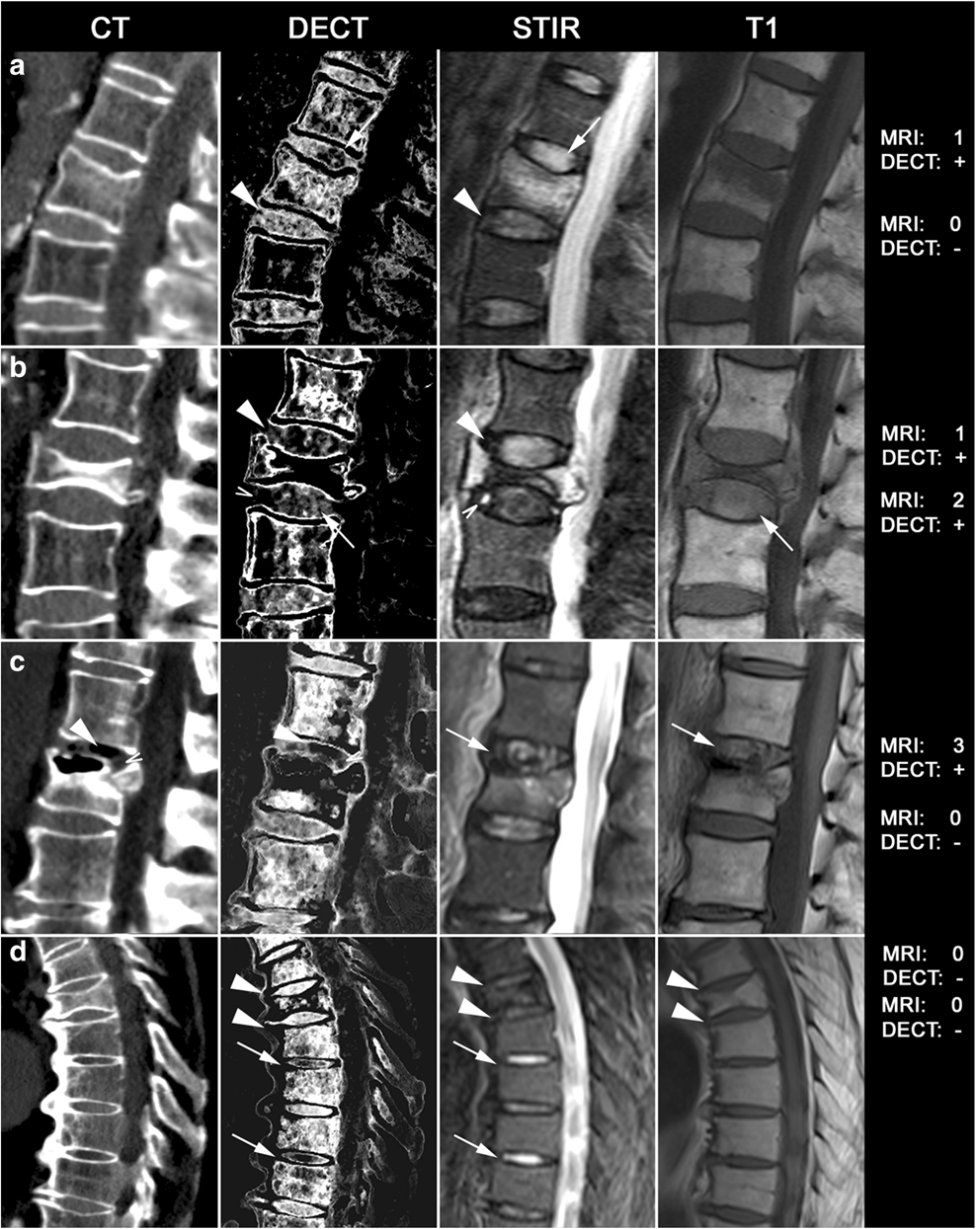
Imaging examples from various patients. Computed tomography (CT), dual-energy collagen maps (DECT), and magnetic resonance imaging short tau inversion recovery (MRI-STIR) and T1 images with combined scores across the three readers for MRI (0–3) and DECT (positive [+] or negative [−]). a A 79-year-old woman with a wedge fracture with bone marrow edema at L2. The cranial disk shows increased signal intensities in MRI-STIR with loss of the nuclear cleft in the posterior aspect (Sander grade 1) and a corresponding loss of collagen density in DECT, especially in the posterior part (arrow), whereas the caudal disk appears normal (arrowhead). b A 78-year-old man with a vertebral compression fracture with bone marrow edema at L2. Again, the cranial disk gives increased STIR signal intensity (grade 1) with a corresponding loss of collagen density in DECT (arrowhead). The caudal disk shows increased T1 signal intensity in the posterior aspect (and a corresponding loss of density in the DECT collagen map; arrow) and focal STIR hyperintensity in the anterior aspect, indicating rupture of fibrous annulus fibers accompanied by even greater signal loss in the DECT (open arrow). c A 66-year-old woman with an incomplete burst fracture at L3. The cranial disk shows high STIR signal, high T1 signal (arrow), and herniation through the endplate in CT (Sander grade 3). The DECT collagen map shows loss of density in areas with air (arrowhead) but also inside the disk material (open arrow). d A 68-year-old man with a wedge fracture at Th7. Adjacent disks show fairly normal signals in STIR and T1 without loss of collagen density in DECT (arrowheads). However, distant disks, not included in the analysis, show high STIR signal intensities (grade 1) and marked decreases in collagen density in DECT (arrows)
